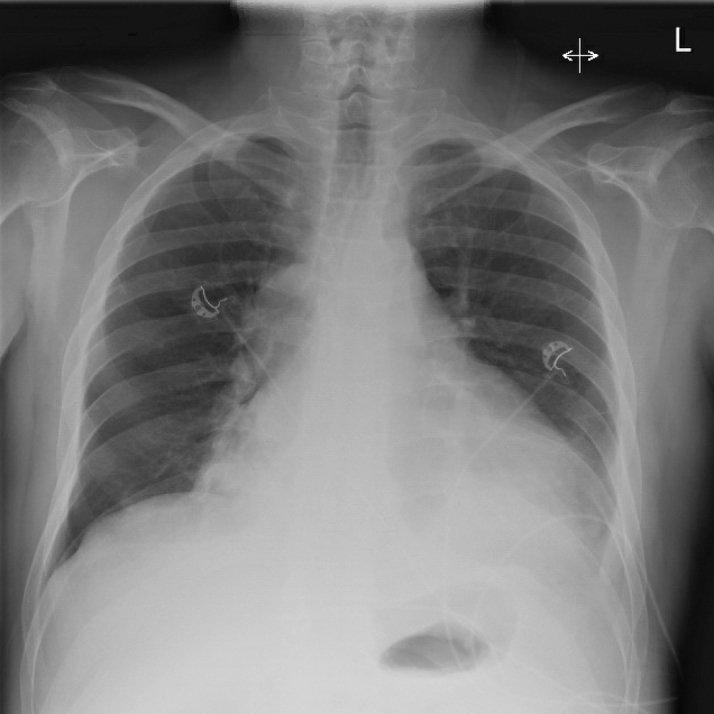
Chest X-Ray Showing an Increased Cardiac Silhouette With a Globular-Shaped Heart
